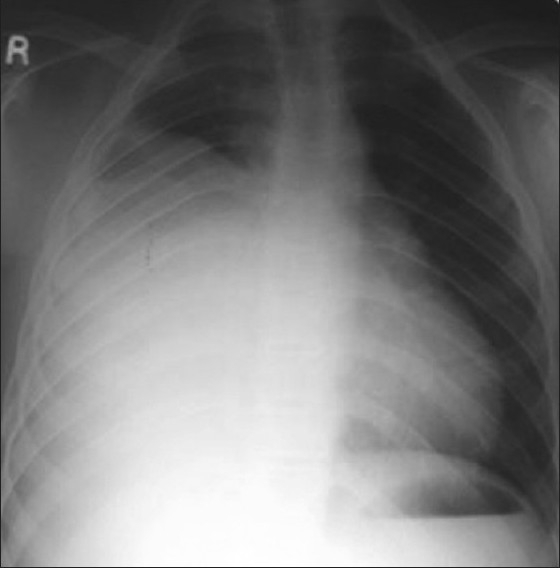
X-ray chest showing anterior mediastinal mass
